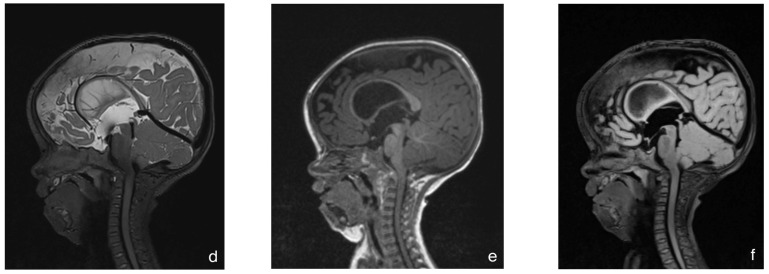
MRI of an 8-month-old child with achondroplasia. (a) Sagittal T2-weighted magnetic resonance image (MRI) shows foramen magnum stenosis with normal signal in the cervical spinal cord. (b) Sagittal T2-weighted MRI highlights a reduced dens–opisthion distance, with the anteroposterior diameter at the C2–C0 level measuring 9 mm. (c) Axial T2-weighted MRI shows ventriculomegaly. (d) Sagittal T2-weighted SPACE image demonstrates reduced perimedullary cerebrospinal fluid (CSF) flow void signal at the cervicocranial junction. (e) Sagittal T1-weighted image shows no evidence of cerebellar tonsillar herniation. (f) Sagittal T2-weighted FLAIR image shows no signs of cervical myelopathy.
